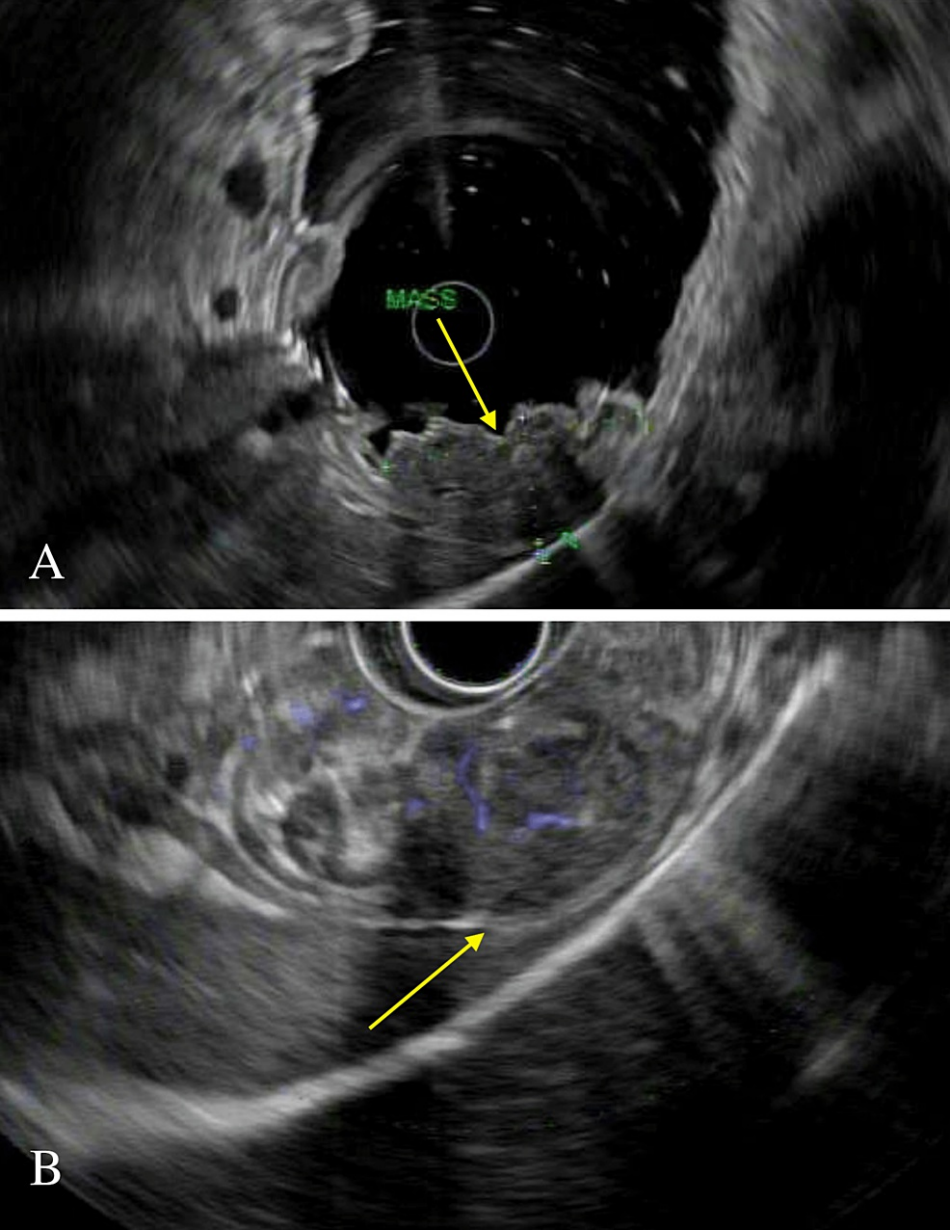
Endoscopic ultrasound showing a fundal subepithelial lesion (A) EUS showing an isolated hypoechoic mass in the fundus without invasion of adjacent serosa, spleen, or diaphragm and (B) Doppler evaluation without evidence of blood flow, excludes varix.
